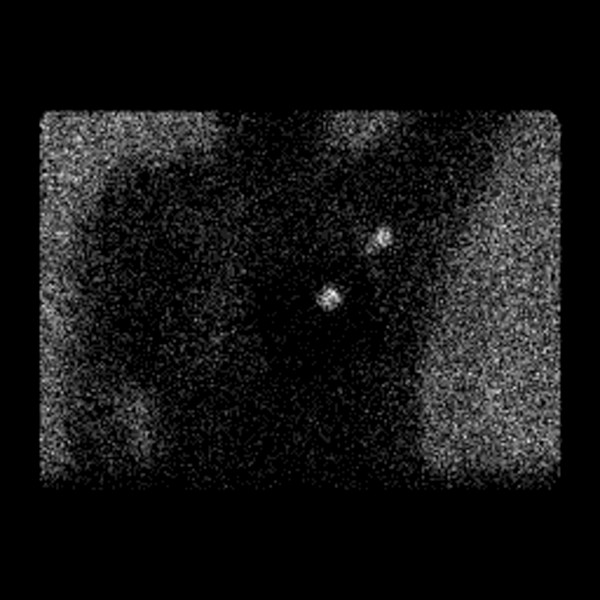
Lymphoscintigraphy. Increased uptake demonstrated at site of injection and at sentinel nodes.
